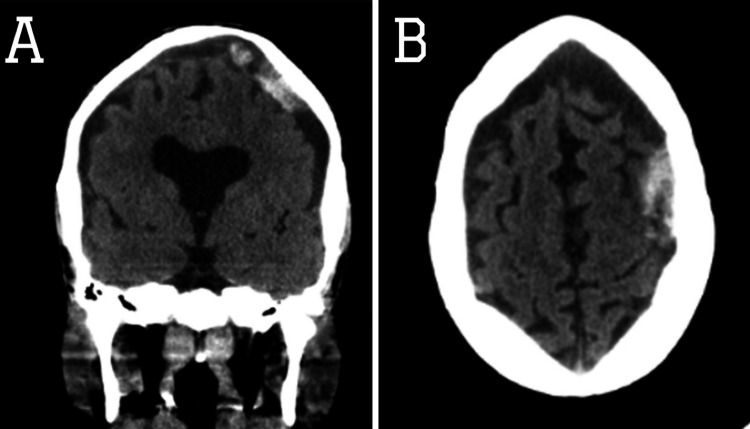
Non-contrast CT scan of the brain Non-contrast CT scan of the brain showing improvement in the size of the left frontoparietal subdural hematoma in both (A) coronal cut and (B) axial cut.
